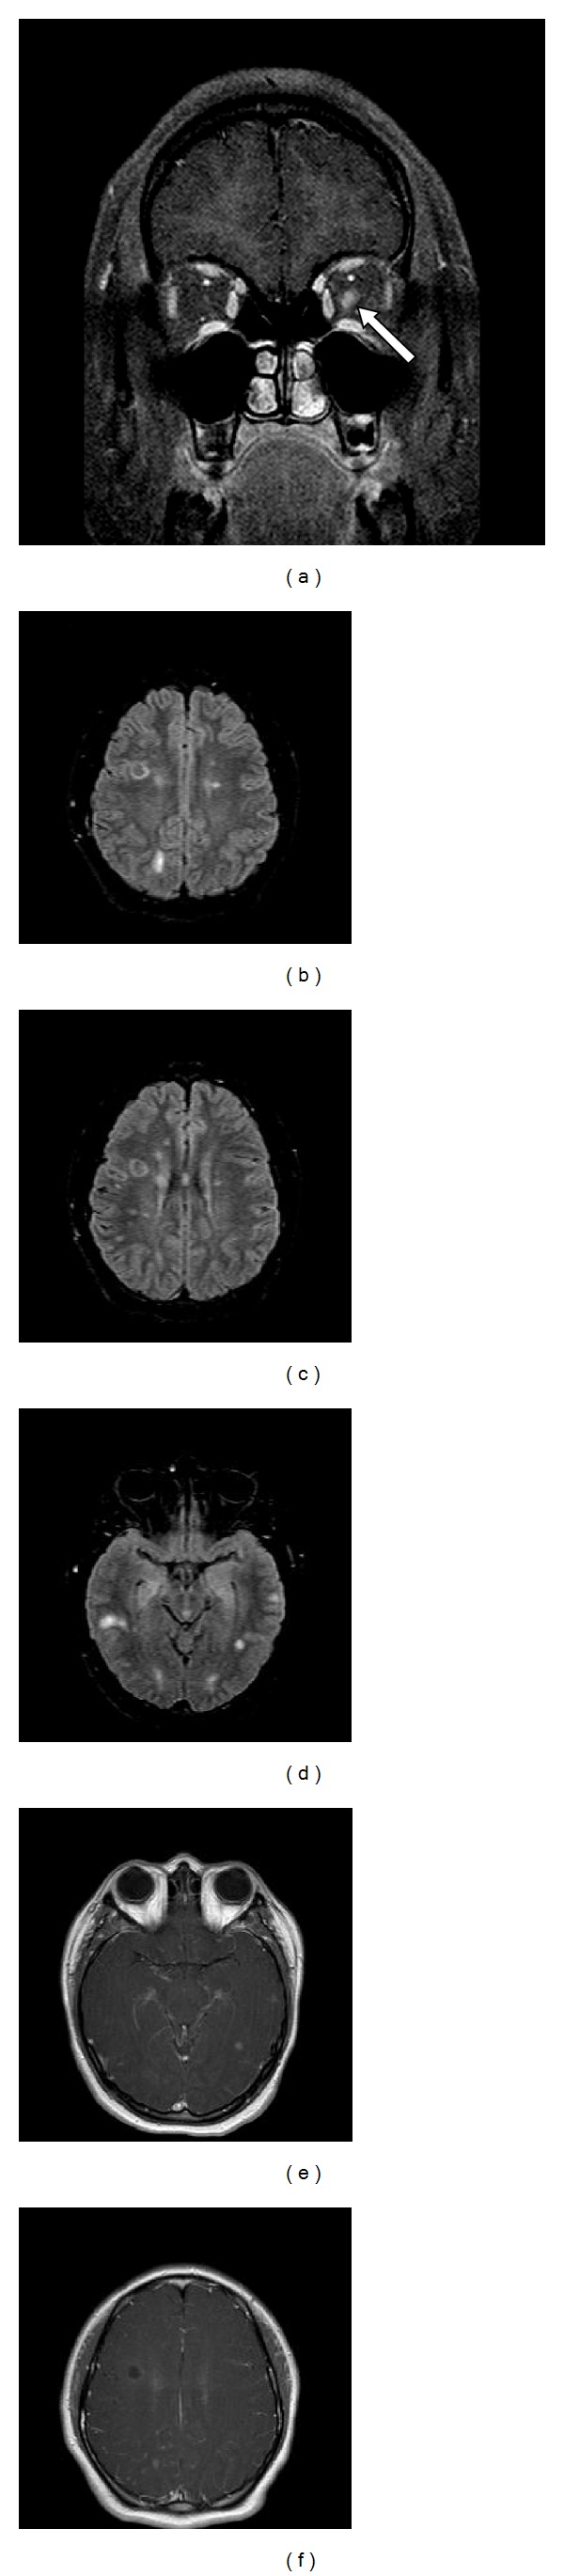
(a) Coronal T1 gadolinium enhanced sequence demonstrating left optic neuritis with enhancement and enlargement of the left optic nerve (arrow). (b) and (c) Axial FLAIR demonstrating typical well-circumscribed ovoid lesions in the juxtacortical and periventricular regions consistent with 2010 McDonald criteria for dissemination in space. (d) and (e) Axial FLAIR and gadolinium enhanced sequences with corresponding asymptomatic enhancing and nonenhancing lesions consistent with 2010 McDonald criteria for dissemination in time. (f) Axial T1 sequence with hypointense lesion associated with acute demyelination and axonal injury.
